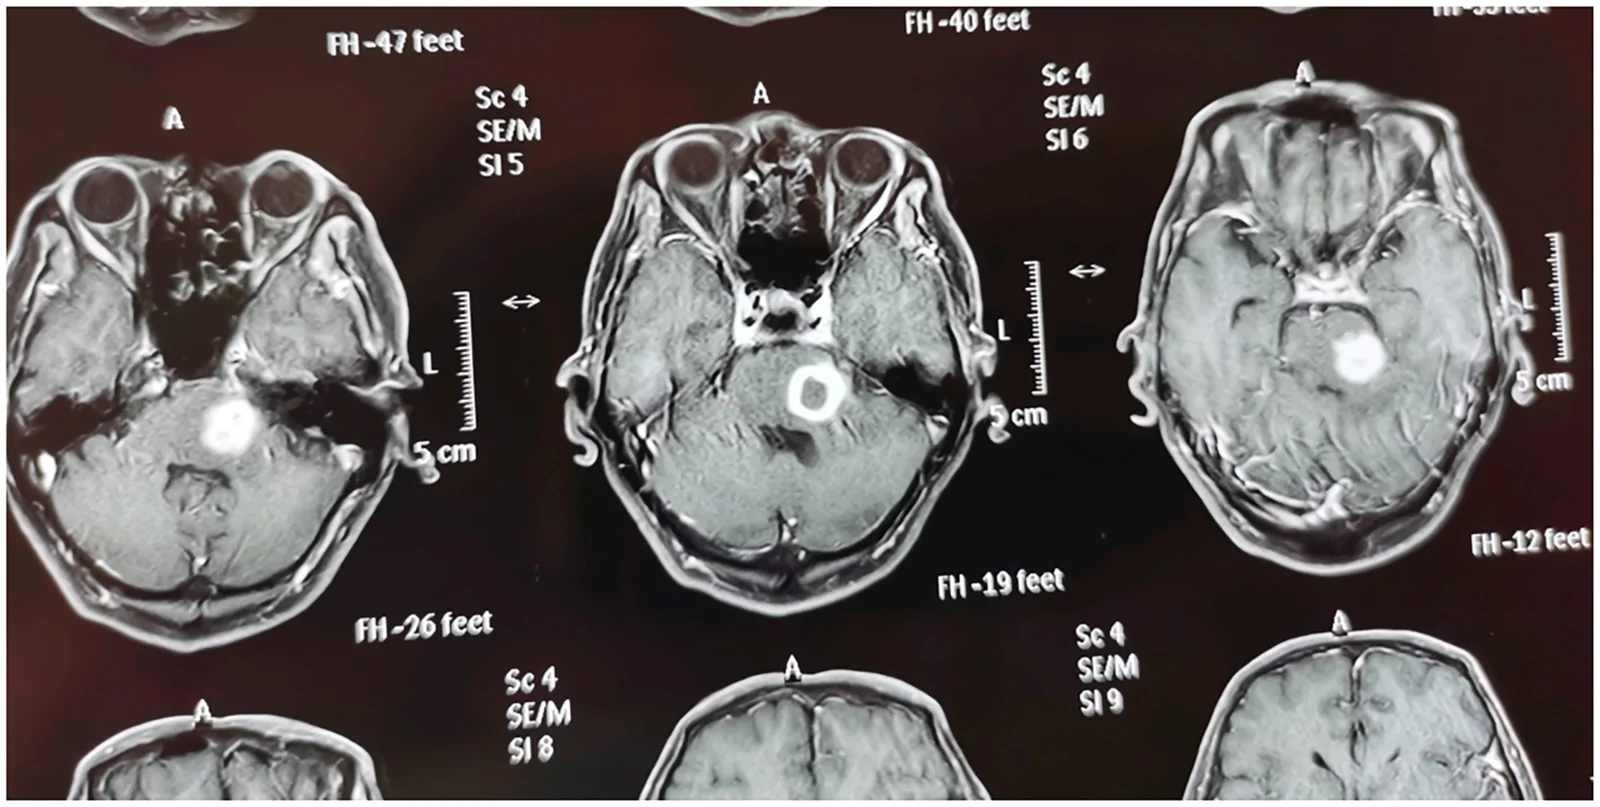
Brain MRI (plain + contrast-enhanced). Location: left pons. Signal characteristics: T1-weighted: isointense (isointense to brain parenchyma); T2-weighted: hypointense; DWI/FLAIR: mildly hypointense. Perilesional edema: prominent. Enhancement pattern: irregular enhancement without peripheral enhancement. MRI = magnetic resonance imaging.
